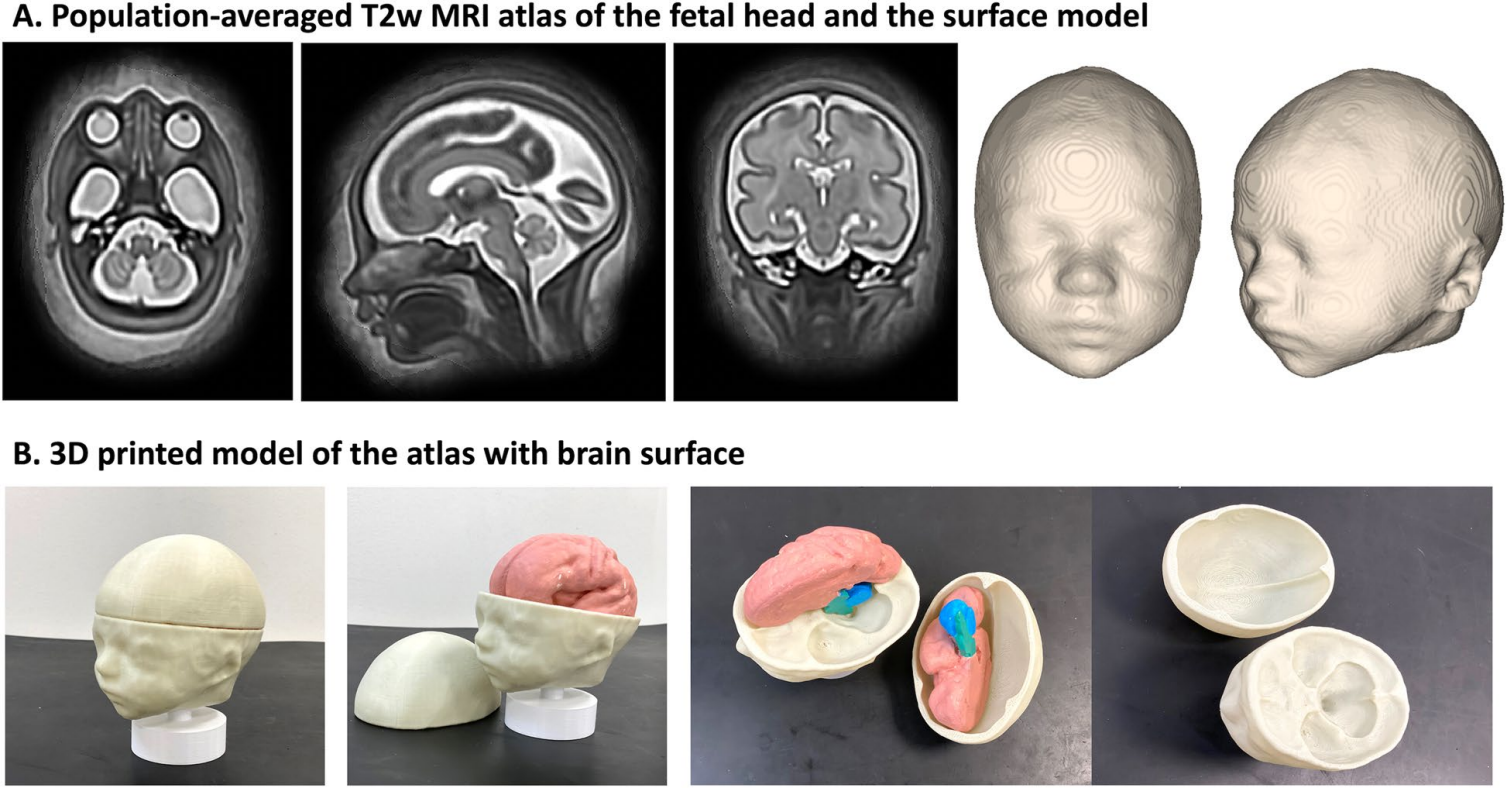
3D population-averaged fetal head MRI atlas and the corresponding surface segmentation (A) and 3D printed physical model (B) with additional brain surface
